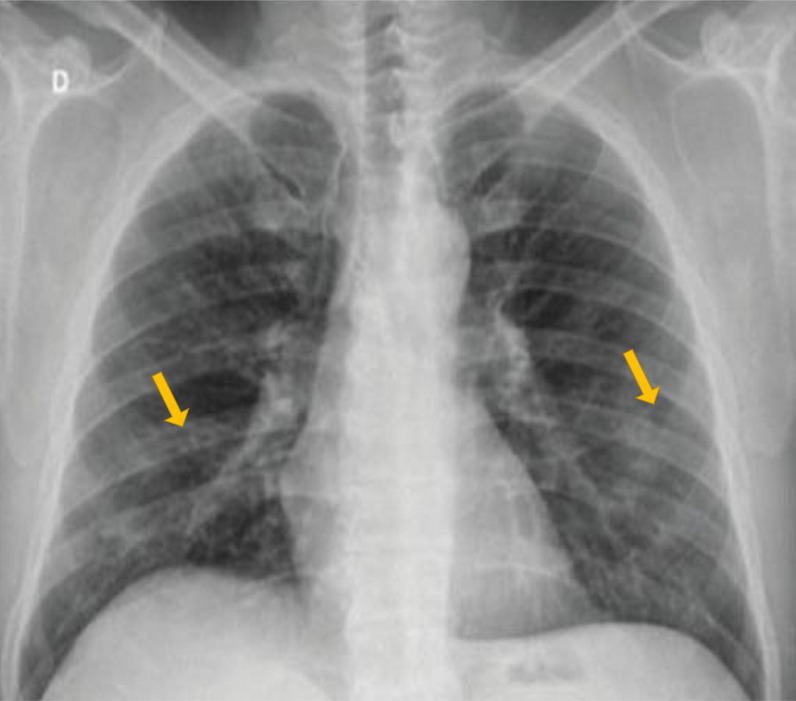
Grade 1 chest X-ray: Alveolar consolidation either unilaterally or bilaterally. Example shows bilateral peripheral alveolar consolidation in the lower lobes.
